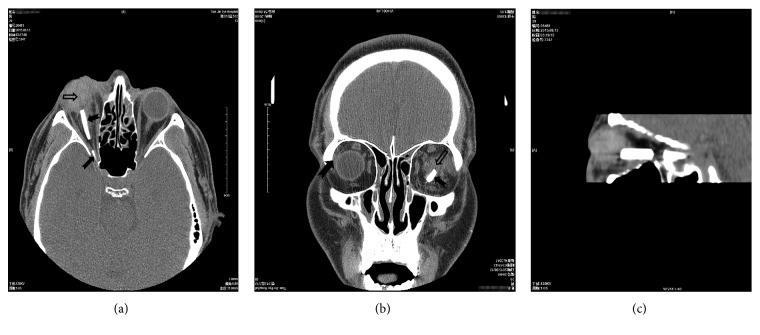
Computed tomography scan of a patient exhibiting eyeball rupture with vitreous hemorrhage (indicated by hollow arrows) and foreign body at the orbital and superior orbital fissure (indicated by solid arrows). (a) Axial scanning. (b) Coronal scanning. (c) Sagittal scanning.
